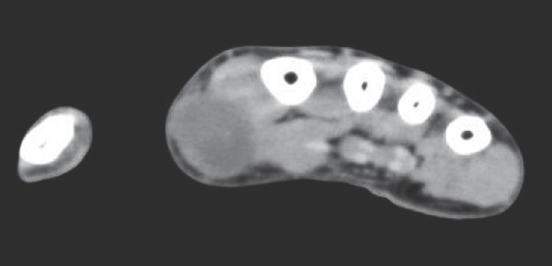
On computed tomography, the 20 mm in diameter mass shows a lower density than the muscle, and its margins are comparatively clear.
